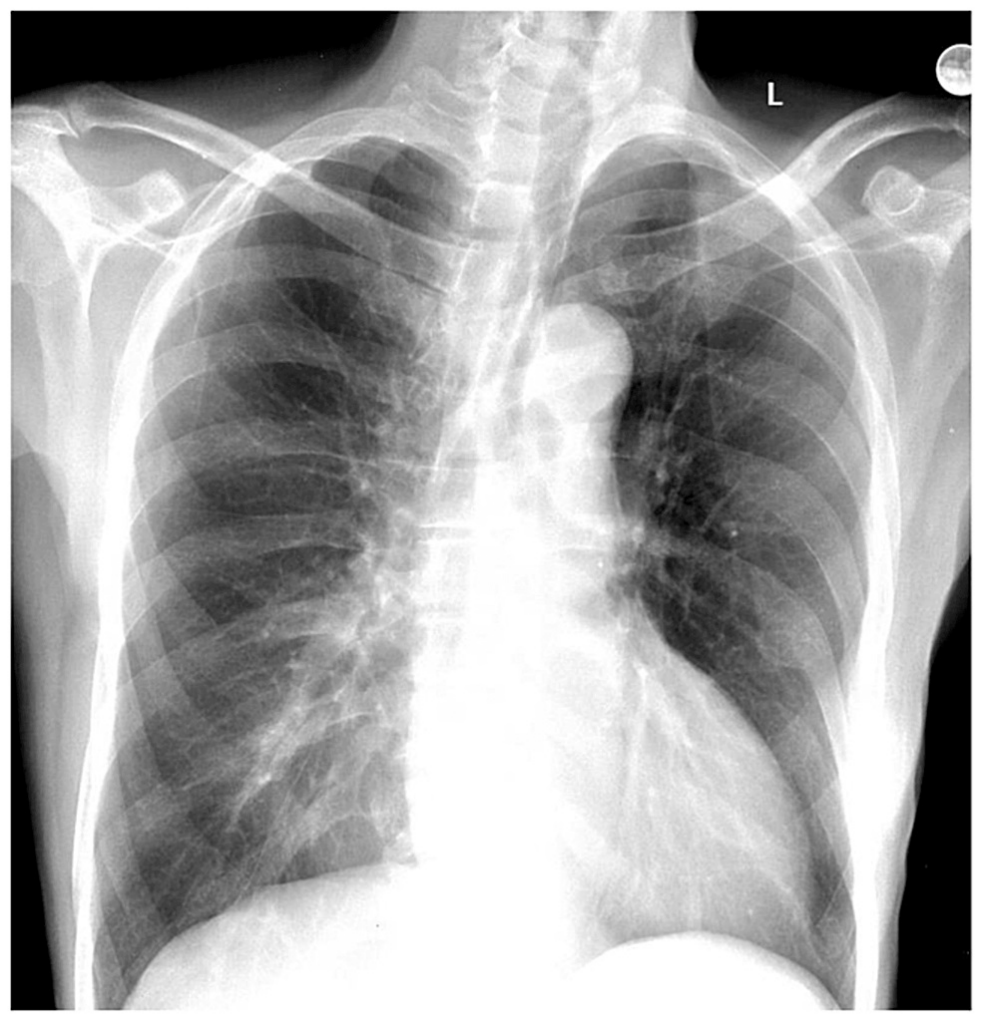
Chest X-ray showing normal appearance
